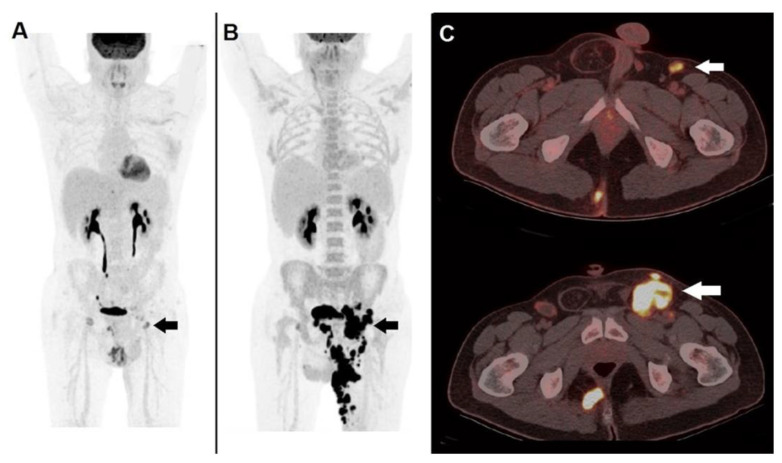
A case of hyper-progression during immunotherapy. A 41-year-old male, affected by non-melanoma skin cancer (locally advanced cutaneous squamous cell carcinoma) of the left thigh, submitted to anti PD-1 immunotherapy (cemiplimab). ^18^F-FDG PET/CT before the start of therapy: (A) MIP image showed pathological tracer accumulation within a left inguinal node (black arrow). PET/CT MIP (B) performed after 2 months of PD-1 blocker depicted an impressive disease progression at nodal and cutaneous level (black arrow). (C) Fused corresponding PET/CT axial (upper row) acquired before therapy well demonstrated a hypermetabolic inguinal node (white arrow), fused PET/CT after 2 months of PD-1 blocker showed meaningful enlargement of the metastatic node (white arrow). Immunotherapy was discontinued, the patient was switched to chemotherapy but deceased after 3 months.
